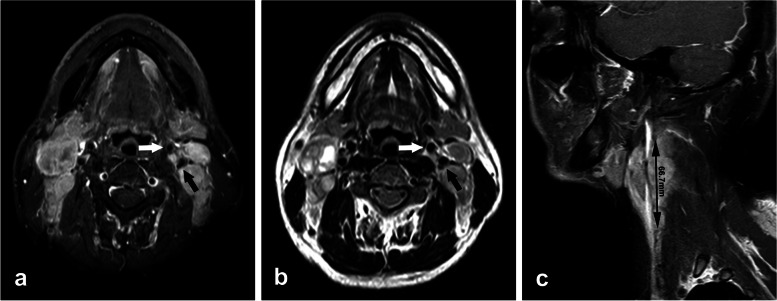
MRI scans of one patient showing lymph node metastases involving the carotid artery. a-b Axial contrast-enhanced T1WI and T2WI showing > 180 degree of encasement of the internal carotid artery with a blurred fat plane (black arrow) and < 180 degree of encasement of the external carotid artery (white arrow) at the left side. c Sagittal contrast-enhanced T1WI showing the length of carotid involvement at the left side, measuring 66.7 mm
